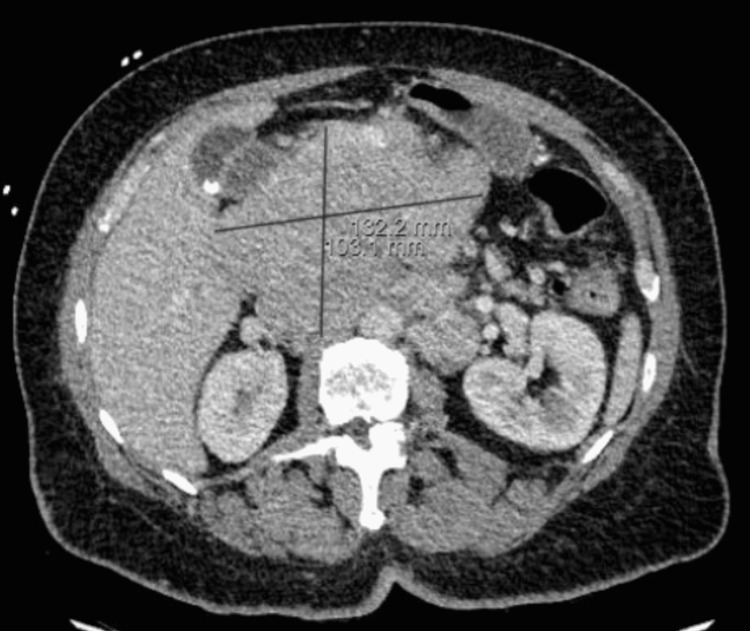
Pancreatic head mass Axial CT scan abdomen and pelvis with IV contrast showing irregular shaped, minimally enhancing solid large pancreatic head lesion measuring 13.2 cm × 10.3 cm
